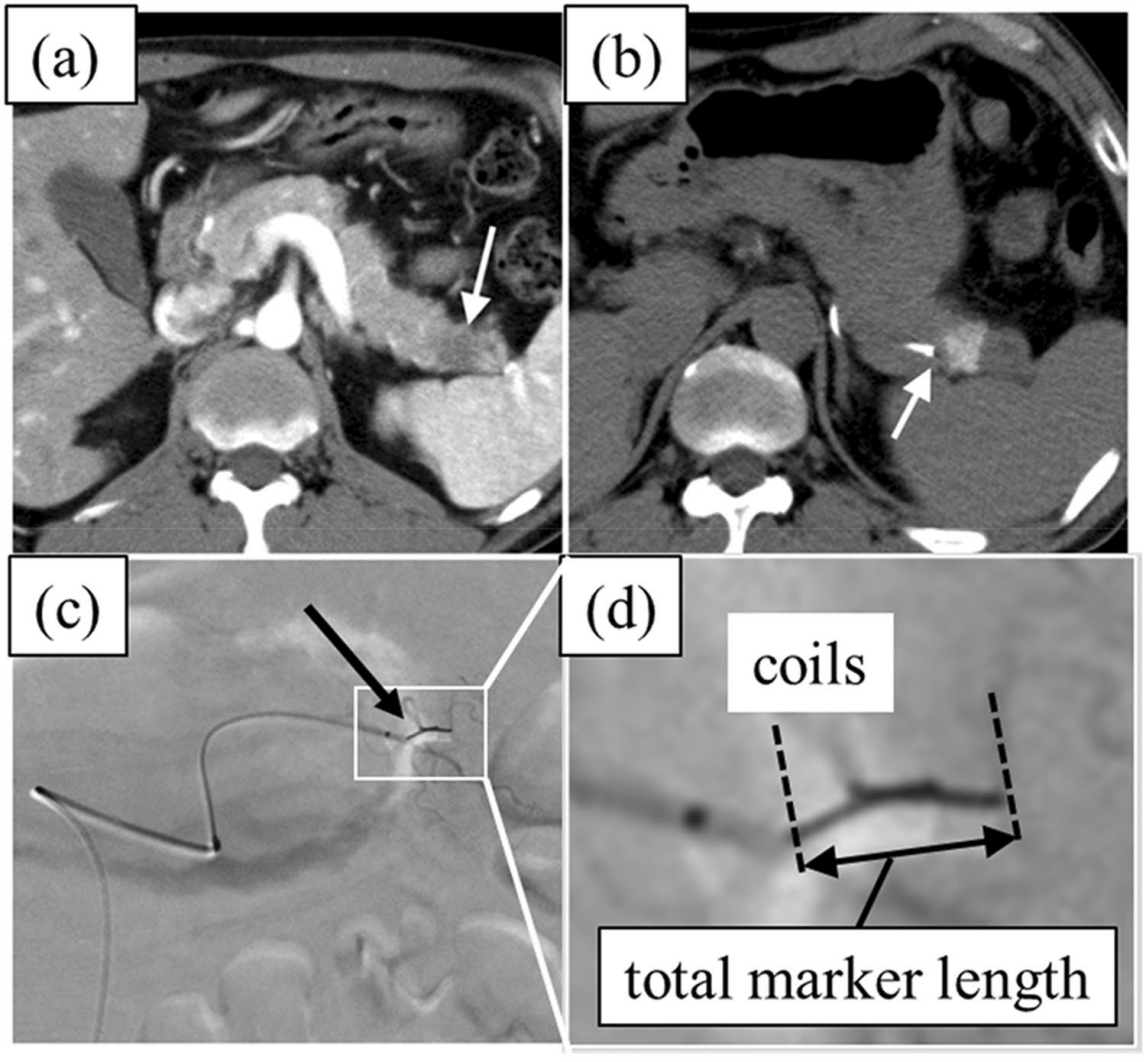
An example case of transarterial marker implantation for pancreatic tail cancer. a Pre-procedural dynamic contrast-enhanced (CE) CT was performed to reveal the tumor location (marked by white arrow). b A microcatheter is inserted into the artery branch near the target cancer and angio-CT is performed to determine the location of the microcatheter tip (white arrow) relative to the target cancer. Residual contrast media injected in the previous angiography also appears on this image. c, d Post-procedural angiogram of the implanted fiducial marker (black arrow; 2 pieces of 5-mm-long straight embolization microcoils with a 0.018-inch diameter)
